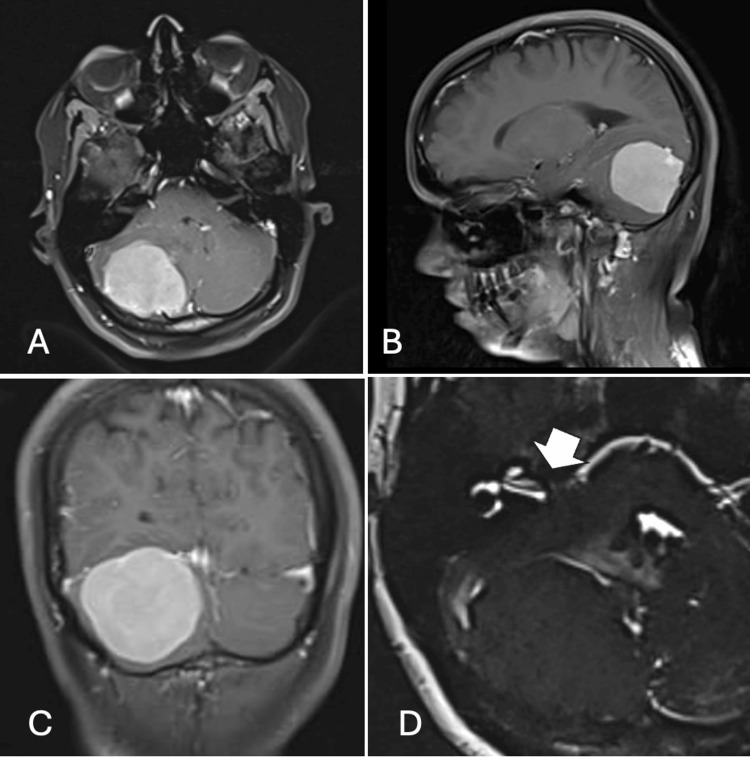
MRI of the brain A) Axial T1-weighted post-contrast image, showing a giant homogeneously enhancing mass in the right cerebellar hemisphere. B) Sagittal post-contrast image, showing convexity attachment of the mass and ventroinferior displacement of the cerebellum. C) Coronal post-contrast image, showing the tentorial attachment of the mass. D) Axial CISS image, showing that the right VII-VIII complex (white block arrow) is kinked and stretched by the flocculonodular lobe of the cerebellum, while the left side is free. CISS: Constructive Interference in Steady State
